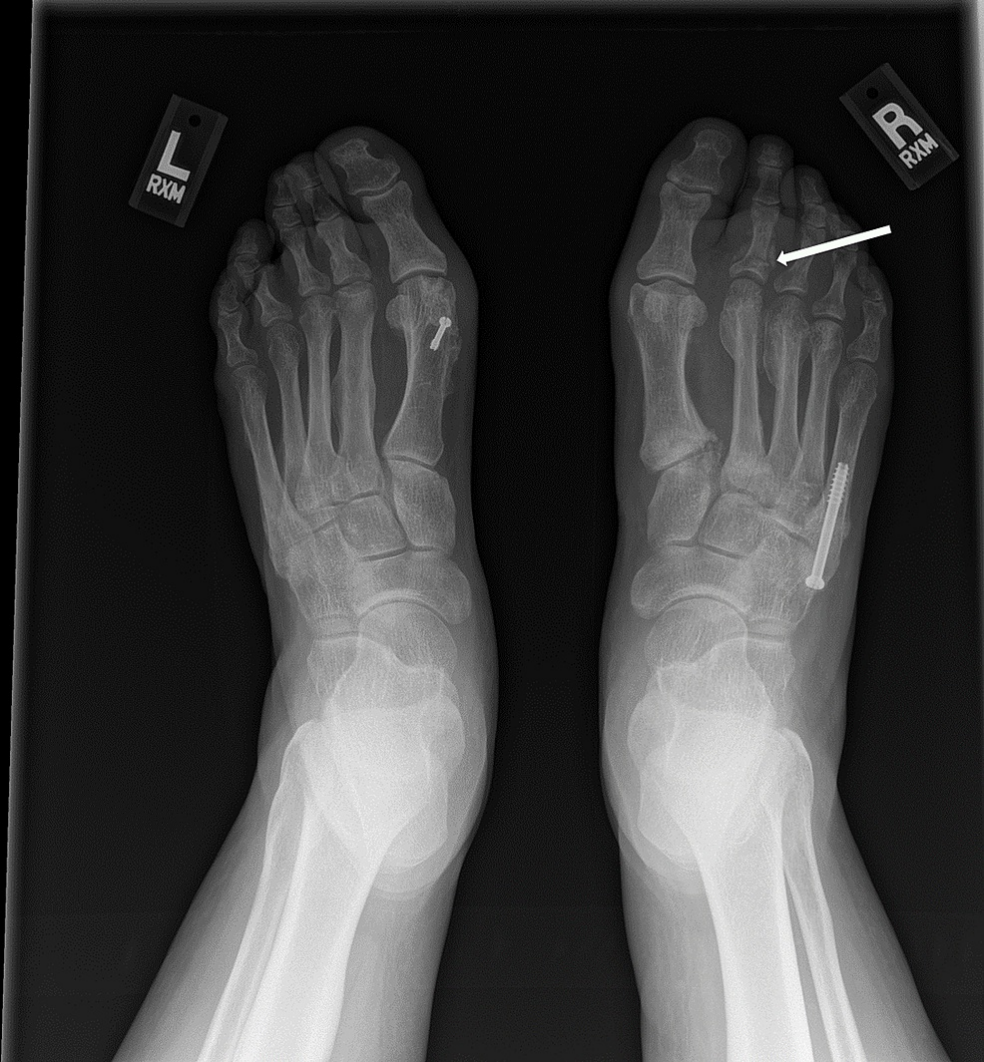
AP radiograph of bilateral feet demonstrating a subacute fracture of the second proximal phalanx of the right foot (arrow).
